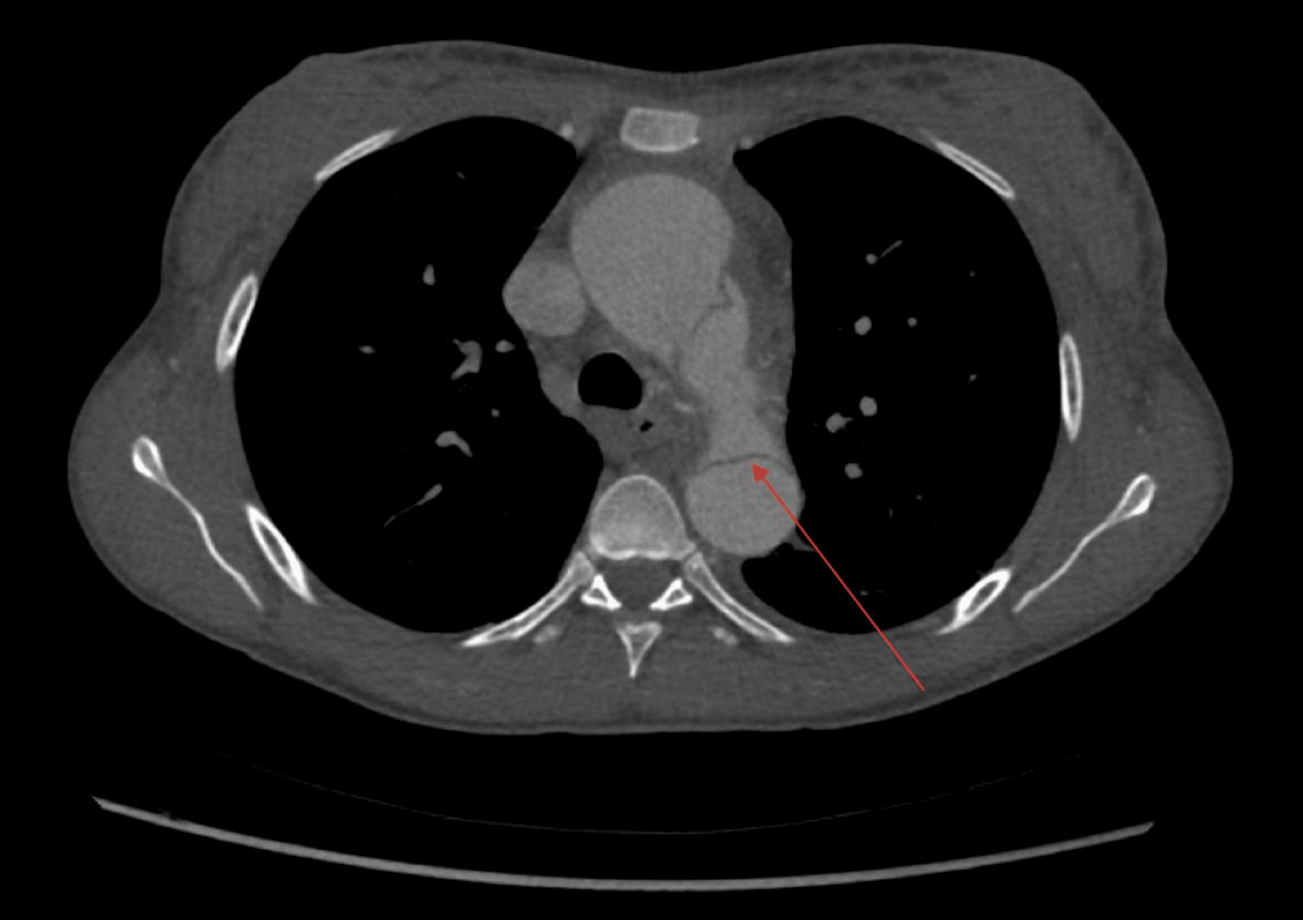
CT scan highlighting dissection flap, also visible is small true lumen and larger false lumen.
